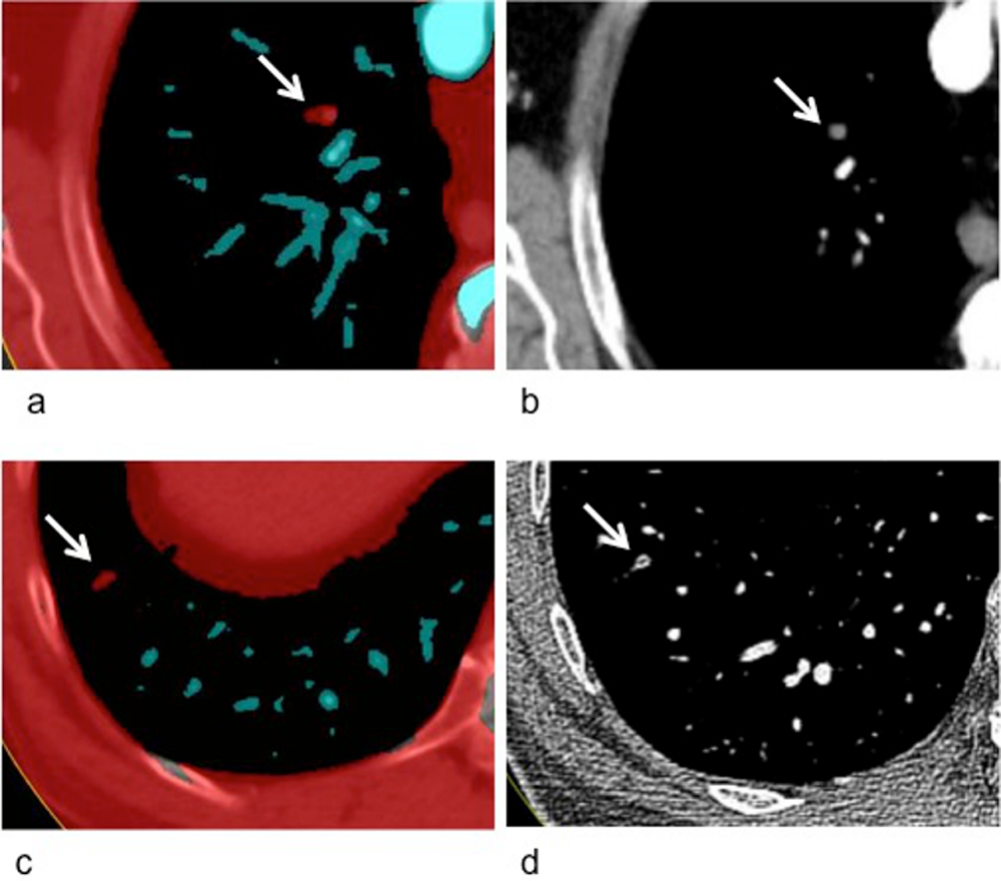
Pulmonary artery embolus in right upper lobe of a male patient (75y), clearly highlighted in dual energy CT (DECT) vessel reconstruction (a), but not detected on grey scale CT (b). Further pulmonary, subsegmental embolus in the right lower lobe of a male patient (77y), highlighted in red in the DECT vessel reconstruction (c). Retrospectively, a filling defect can be seen in thin-slice (1 mm) reconstructions of the corresponding grey scale CT (d).
